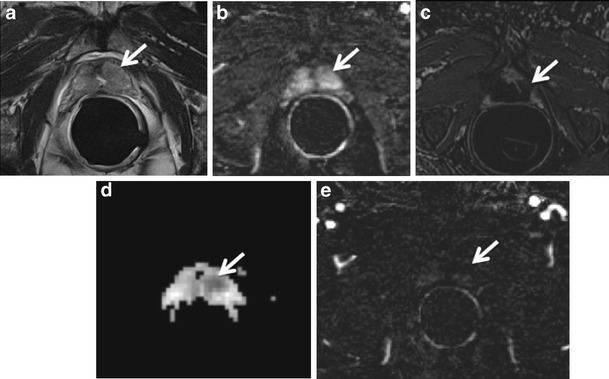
Post-MRgFUS treatment. a Pre-treatment axial T2-weighted fast spin-echo image and b post-contrast subtracted image showing a focal lesion on the postero-lateral aspect of the left third mid-gland with enhancement consistent with prostate cancer. c Post-treatment axial T1-weighted post-contrast subtracted image showing ablation of the area. d ADC map 6 months after MRgFUS treatment showing a focal zone of restricted diffusion suspected for local recurrence. e Post-contrast subtracted image showing no focus of contast enhancement. These findings are consistent with post-MrgFUS treatment fibrotic tissue
